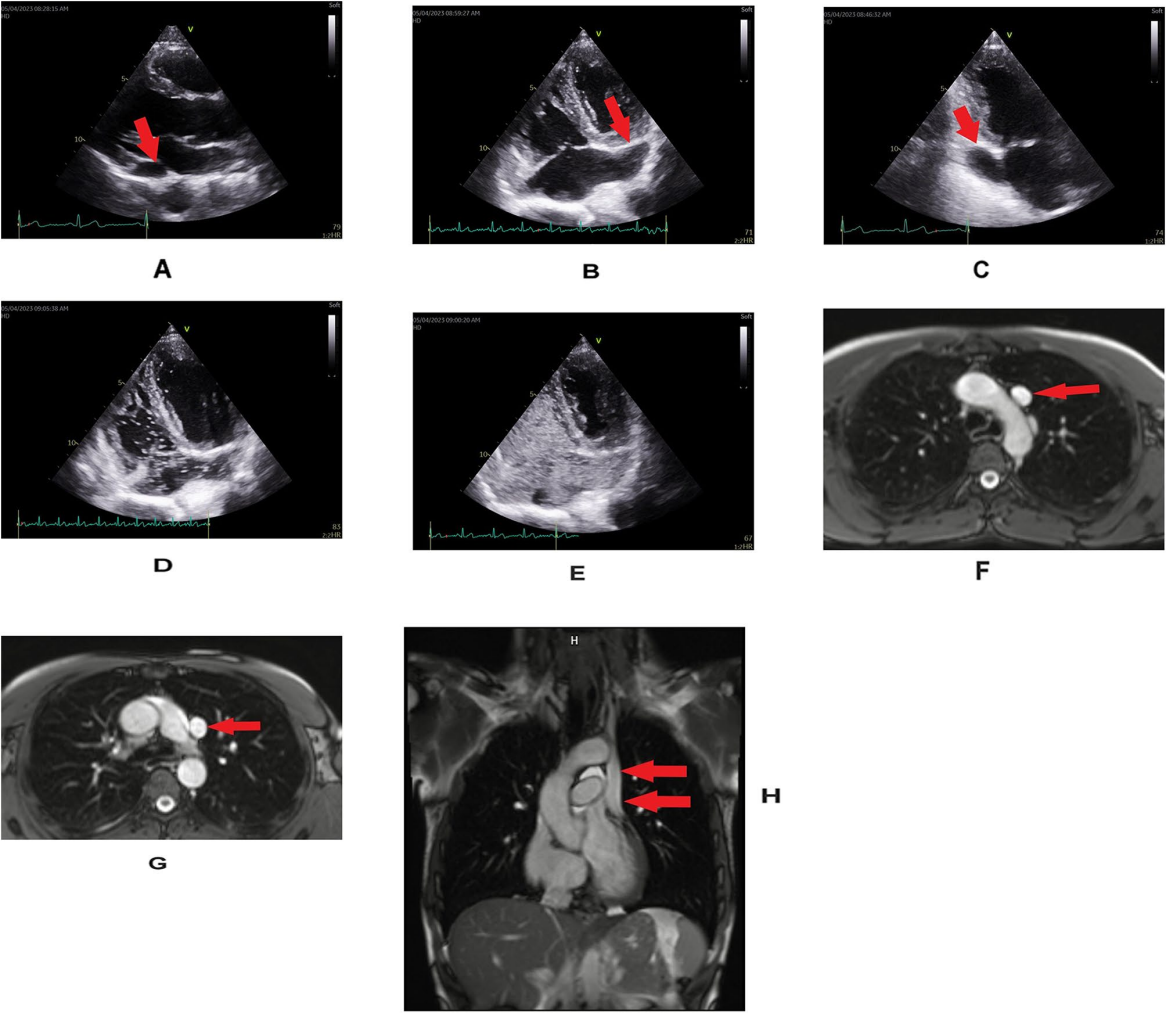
A, B, C, D, E. Transthoracic echocardiography. A, B, C. Significantly dilated coronary sinus (arrows)- dimensions; parasternal long axis view- 2.3 × 1.4 cm (A), four-chamber view- 2.2 cm (B), two-chamber view- 2.9 × 1.7 cm (C). D, E. The injection of agitated saline into the right arm vein resulting in the opacification of a dilated coronary sinus followed by right atrial filling. F, G, H. CMR. Single-shot SSFP images, showing a single persistent left SVC (arrows), with the right-sided SVC missing in its typical position to the right and posteriorly from ascending aorta. F, G. The image from an axial stack. H. The coronal image CMR-cardiac magnetic resonance, SSFP-steady state free precession sequence, SVC-superior vena cava
